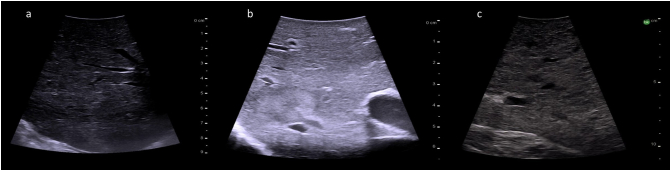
Intraoperative ultrasound images of liver parenchyma. a) Normal liver parenchyma (Frequency 10 MHz), b) Hepatic steatosis (Frequency 10 MHz), c) Cirrhosis (Frequency 5 MHz).
